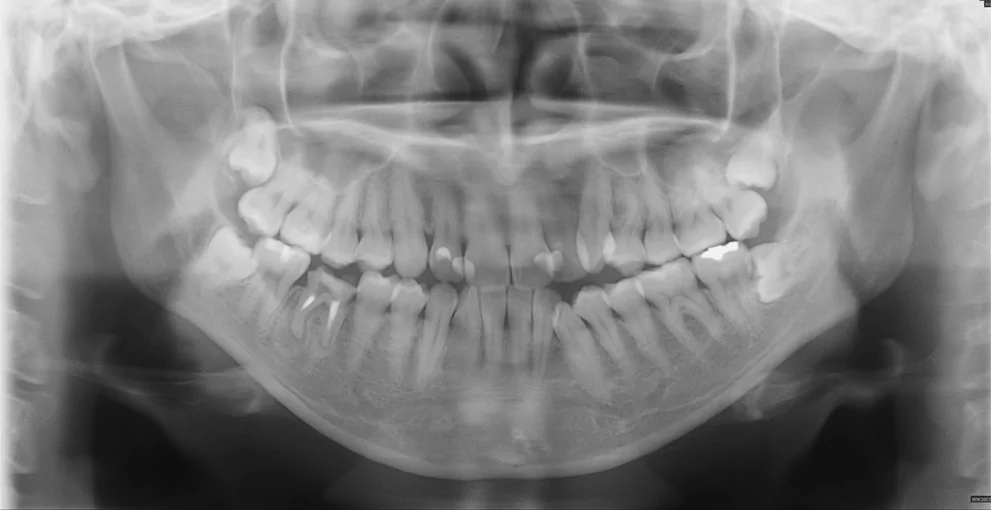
A panoramic radiograph image. Panoramic radiographs taken at the initial visit on December 11, 2023. Enamel pearls cannot be confirmed at the maxillary left third molar site in the left maxillary sinus floor.
